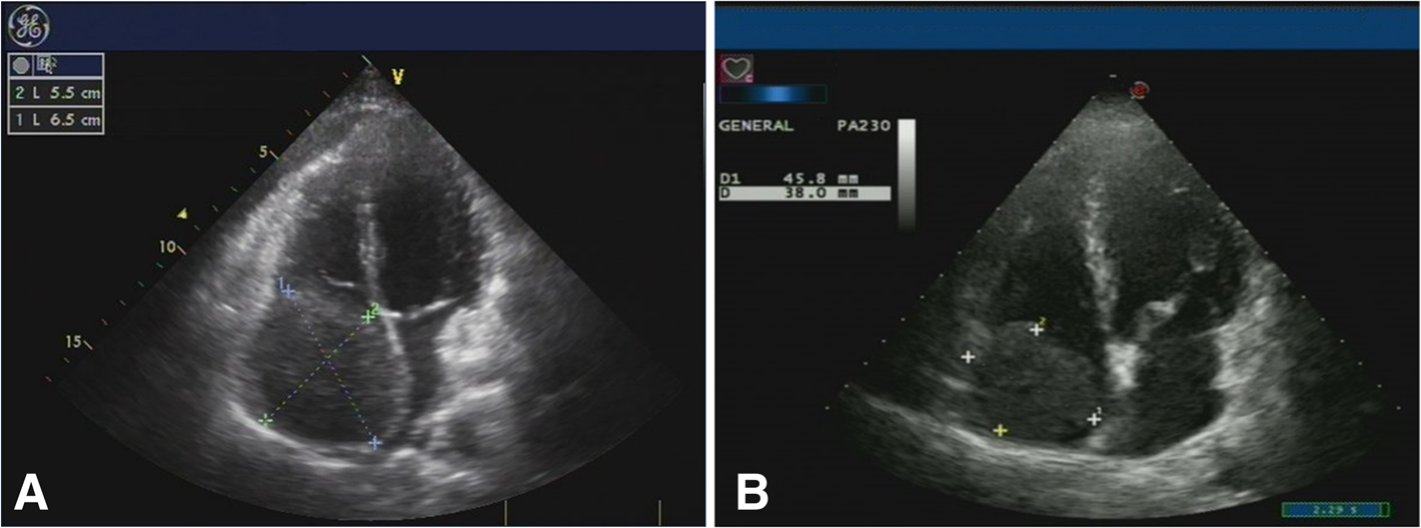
Doppler ultrasound follow-up images of one RA TT case. a Before HFRT, the TT (5.5*6.5 cm) filled the right atrium. b 14 days after completion of RT, the TT (3.8*4.6 cm) was decreased in size
